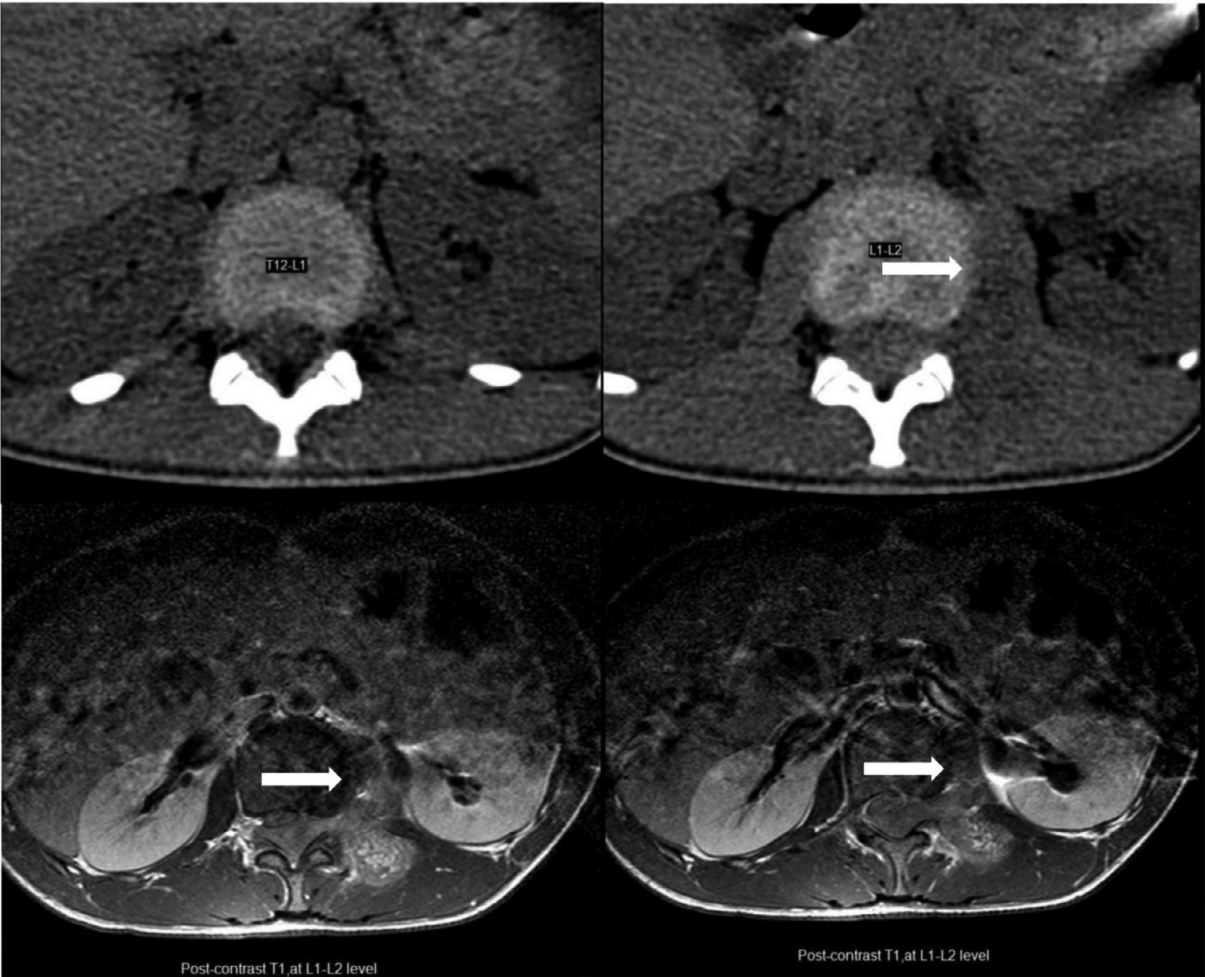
Computed tomography (CT) scan of the thoracolumbar spine shows s mass-like lesion with soft tissue density was seen on the left side of the spinal canal at the L1-L2 level with extension to the neural foramen and the left paraspinal muscles at the same level. White arrows indicate mass lesion.
